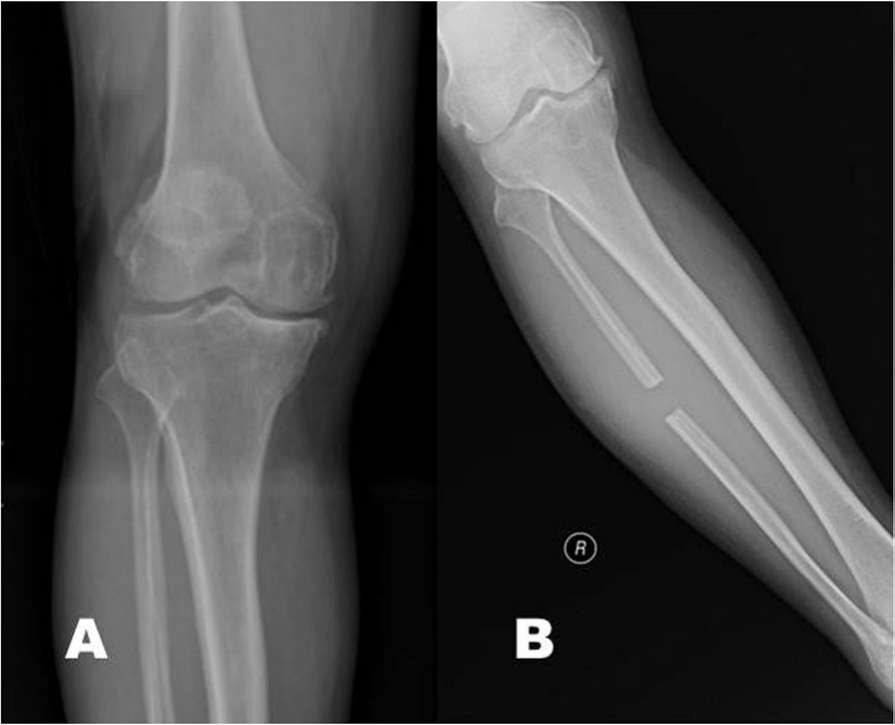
A Preoperative anterior–posterior x-ray. B Postoperative anterior–posterior x-ray: modified partial fibular osteotomy at the level of 15 cm below the fibular head
